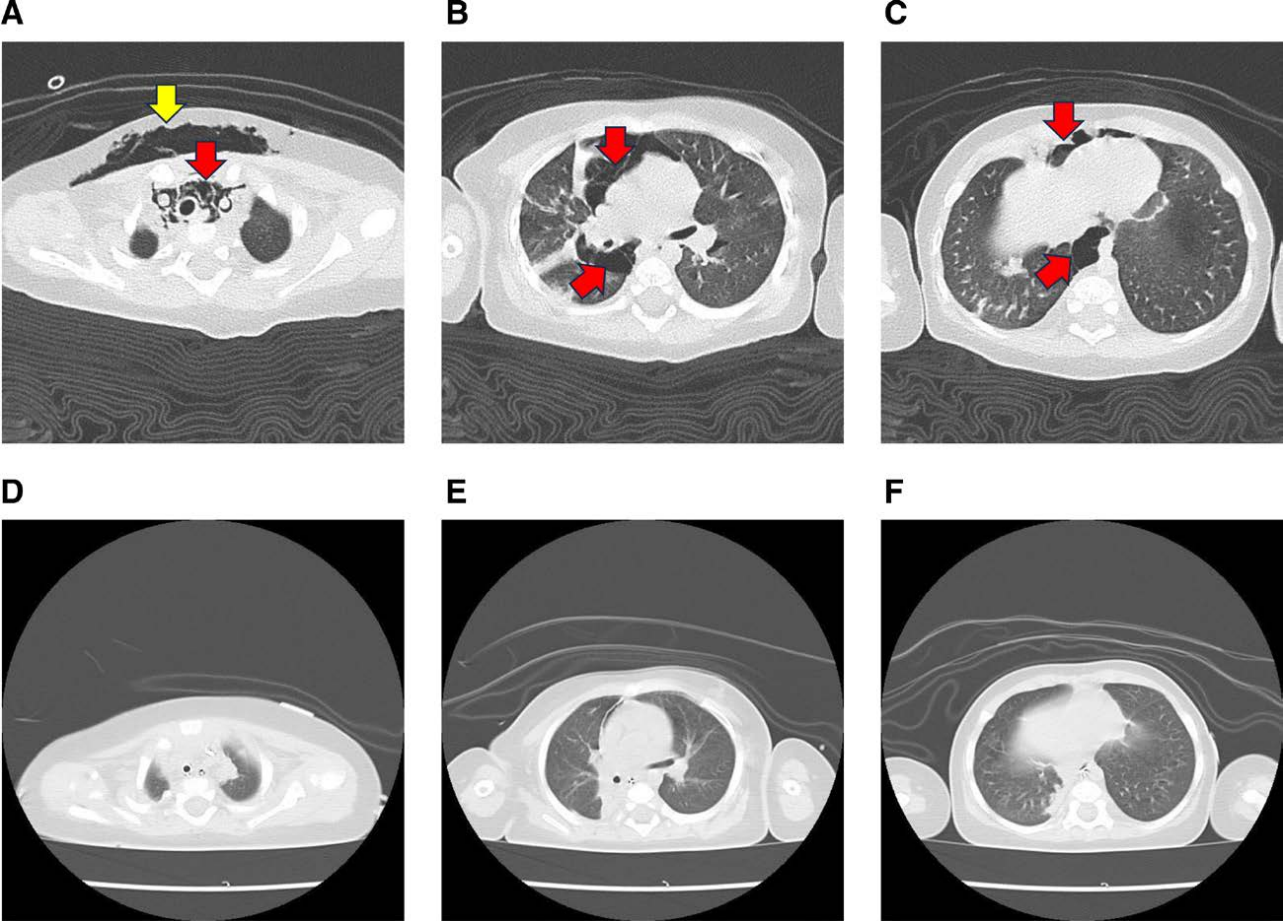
(A–C) Axial CT images on the day of ICU admission showed severe pneumomediastinum (red arrow) and subcutaneous emphysema (yellow arrows). (D–F) Axial CT images on the 5th day of ICU admission demonstrate the improvement of pneumomediastinum and subcutaneous emphysema. CT = computed tomography, ICU = intensive care unit.
